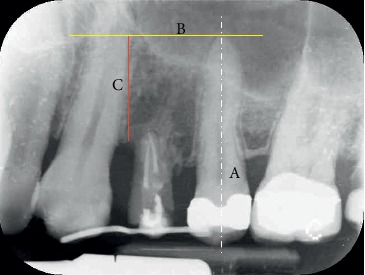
Tooth 2.4: X-ray at T1: the most apical point of the bone defect is marked and its distance (C) from the straight line (B) perpendicular to the long axis of the near tooth (A) and passing through the apex is measured. The difference between the linear measurement T1 and T0 gives the vertical bone gain in mm.
